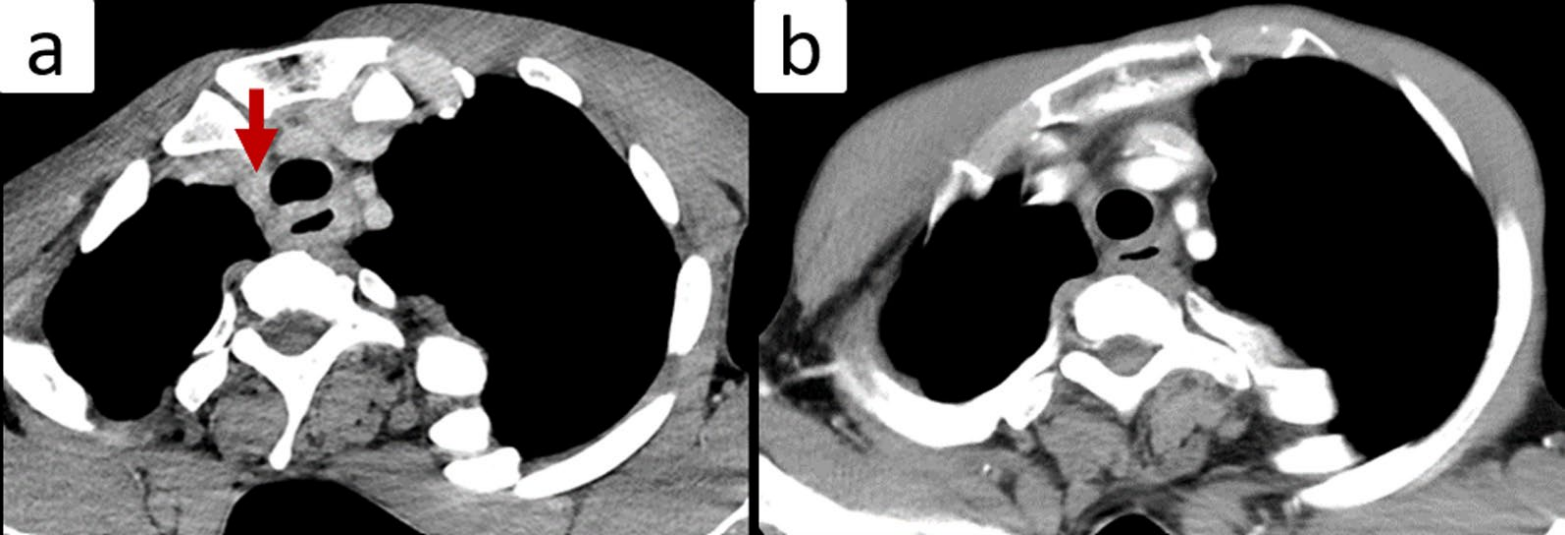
Contrast-enhanced CT images: a Before chemoradiotherapy, there was thickening of the esophageal wall from the upper to middle thoracic esophagus, and enlarged lymph nodes were present around the right recurrent nerve (arrow). b After chemoradiotherapy, CT showed reduced esophageal wall thickening with no enlarged lymph nodes
